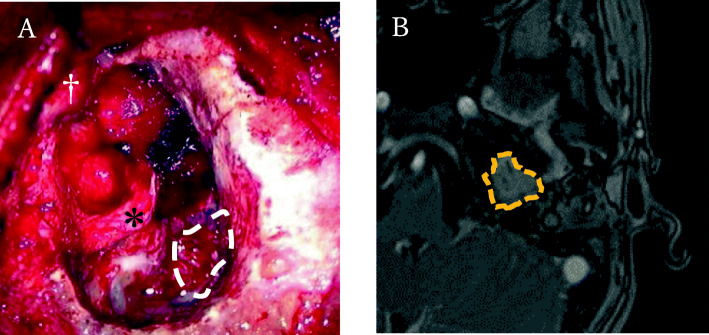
a At the end of the surgical removal. The tumor was spared in the internal auditory canal and the deep layer of the epitympanum (white dotted area: residual tumor in the internal auditory canal). *: facial nerve; †: temporomandibular joint). b MRI at 2 years postoperatively shows a residual lesion of about 2 cm in the deep part of the left petrous bone near the internal auditory canal up to the epitympanum (dotted area), which did not show regrowth compared to the MRI images at 2 months after the surgery
